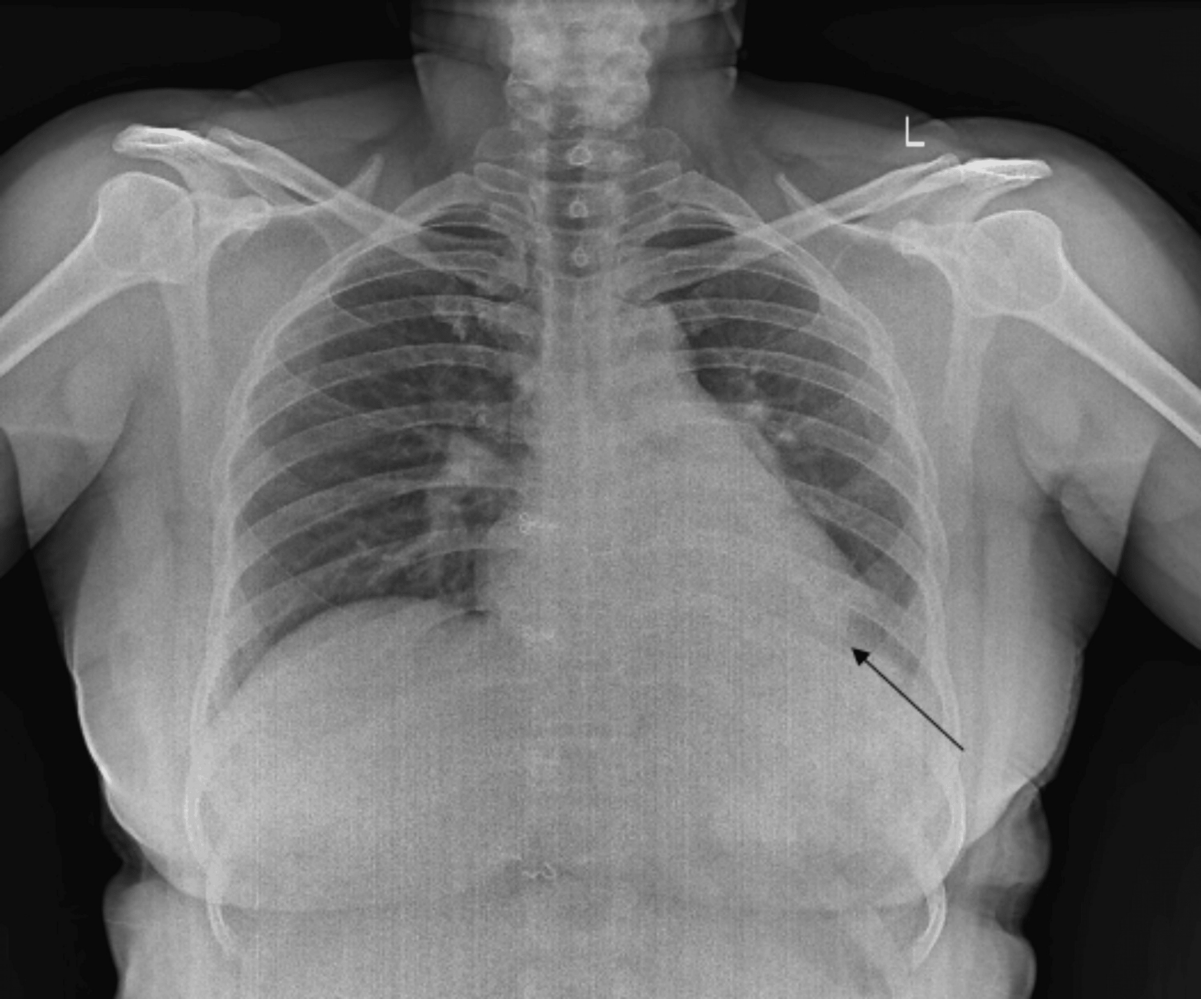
Chest radiograph reveals a suspicious collapse of the left lower lobe, indicated by a black arrow.
